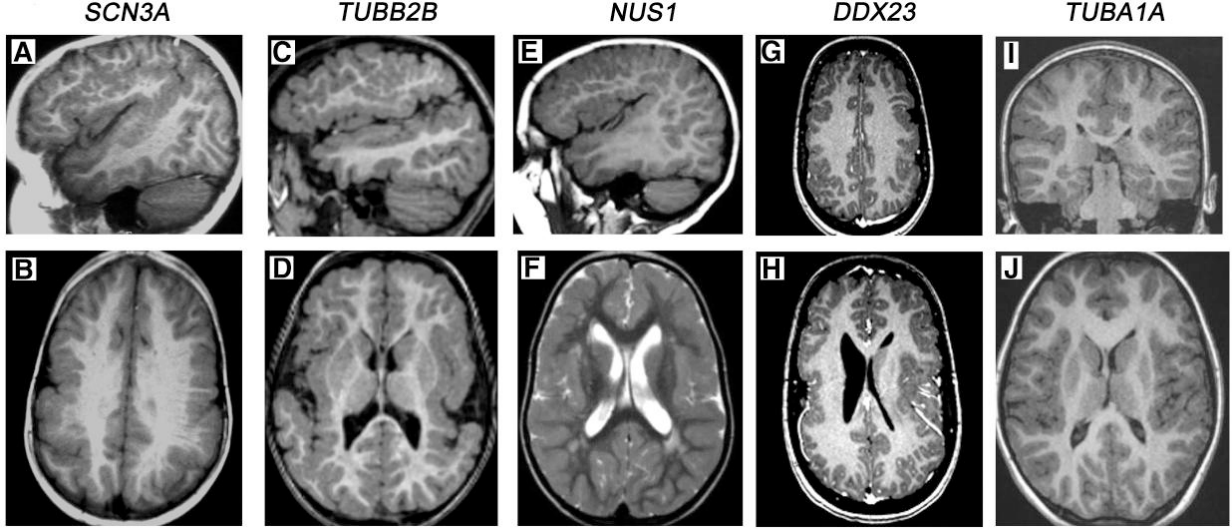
Brain MR images of patients with pathogenic or likely pathogenic gene variants. The subject with an SCN3A variant shows BPP and thick grey matter over the frontal, parietal and perisylvian cortexes (A, B). The subject with a TUBB2B variant has bilateral fronto-parietal, perisylvian and insular polymicrogyria (C, D). The subject with an NUS1 variant has a similar pattern of distribution of polymicrogyria as seen with SCN3A and TUBB2B but additional white matter changes around the posterior horn of the lateral ventricles (E, F). The subject with a DDX23 variant has asymmetric patches of frontal, parietal and insular/perisylvian polymicrogyria (G, H). The polymicrogyric cortex of the subject with a TUBA1A variant is limited to the opercular and insular cortexes (I, J). SCN3A, the sodium voltage–gated channel alpha subunit 3; TUBB2B, tubulin 2B; NUS1, dehydrodolichyl diphosphate synthase subunit; *DDX23*, DEAD-box RNA helicase 23 gene; TUBA1A, tubulin alpha-1A.
